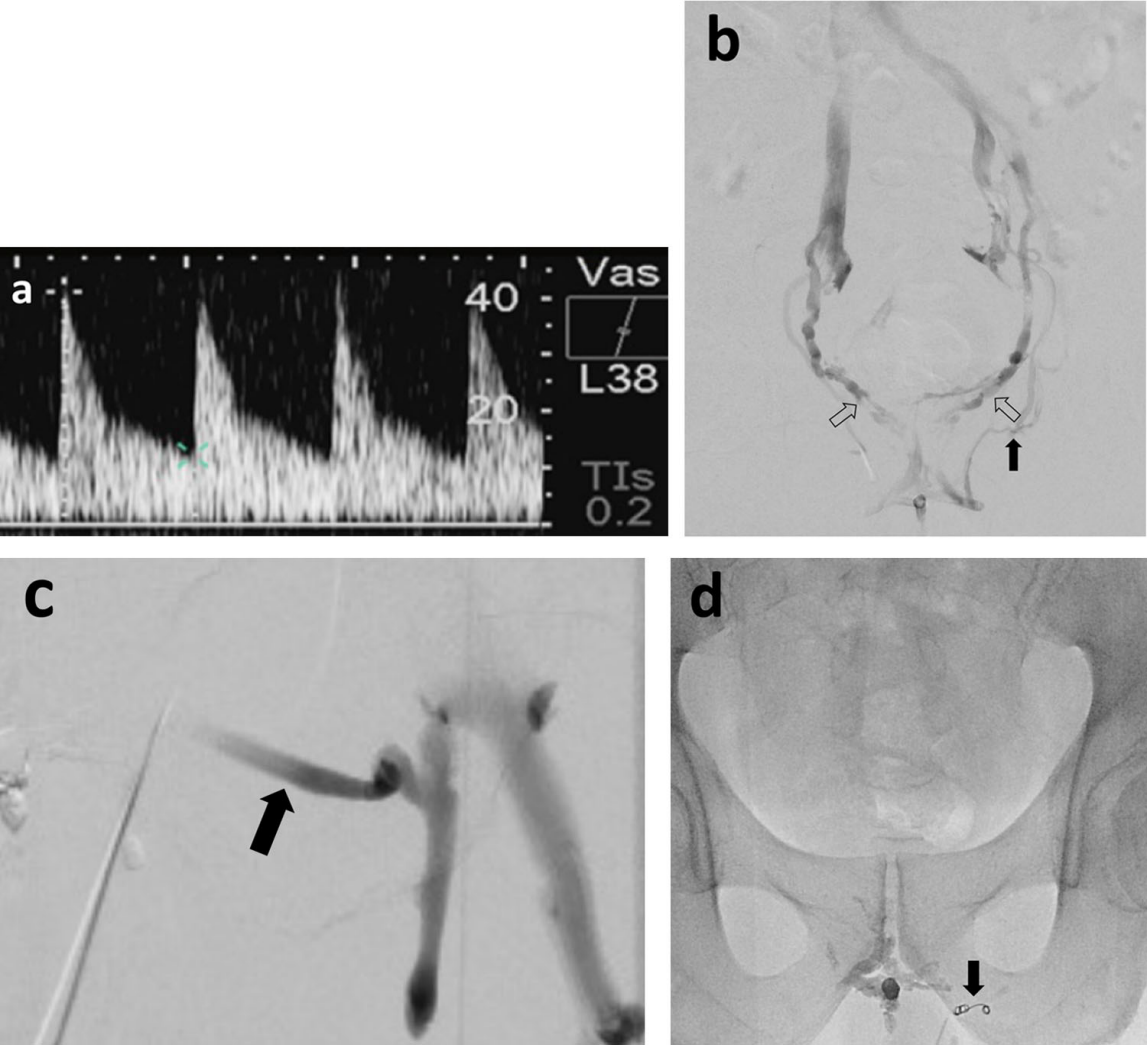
72-year-old patient with severe erectile dysfunction not responding to PDE-5-inhibitors. Penile duplex sonography demonstrated increased end-diastolic flow velocities a. Venogram b demonstrating venous leak of internal (open arrows) and external (arrow) pudendal veins with left external pudendal vein (arrow) draining in the left saphenous vein c. Embolization was performed using Glubran-2 after protective coil embolization of the left external pudendal vein with coils d
